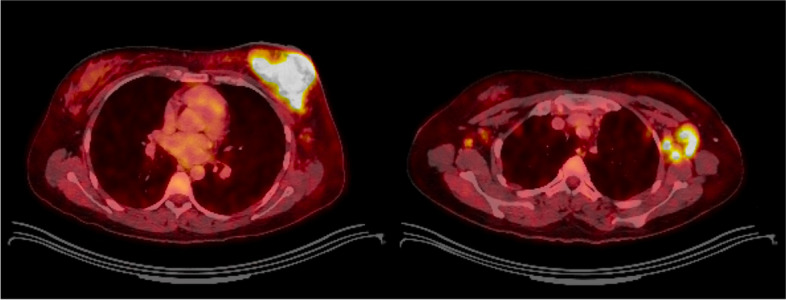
A staging positron emission tomography (PET)/computed tomography (CT) demonstrated a hypermetabolic 9.5 cm multilobulated centrally necrotic left breast mass with (B) extensive hypermetabolic left axillary and retropectoral lymphadenopathy. Nonenlarged hypermetabolic right axillary lymph nodes, were favored to be related to her recent documented COVID-19 vaccine administered in her right deltoid
